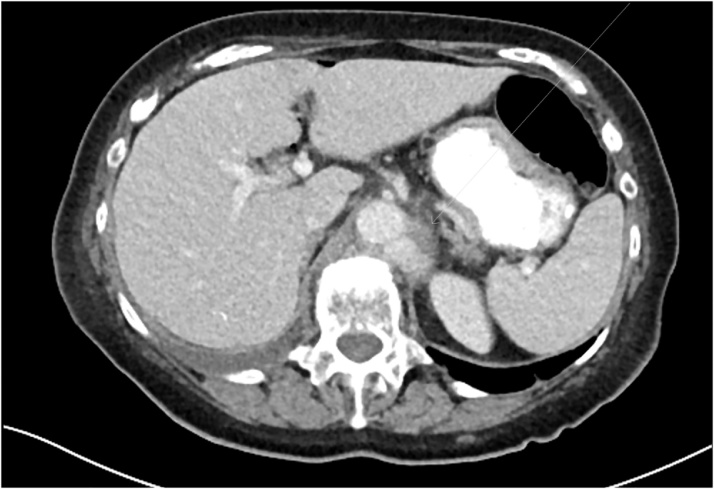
Post-operative transverse CT scan demonstrating mycotic abdominal aortic aneurysm.
